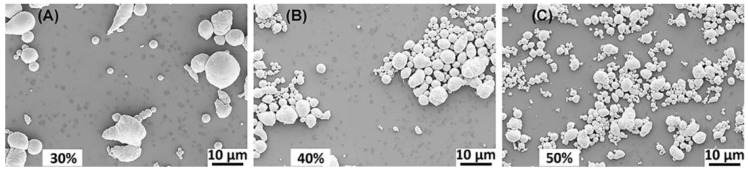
Morphology images of eGaIn nanodroplets under different ultrasonic amplitudes: (A) 30% (B) 40%, and (C) 50%.
